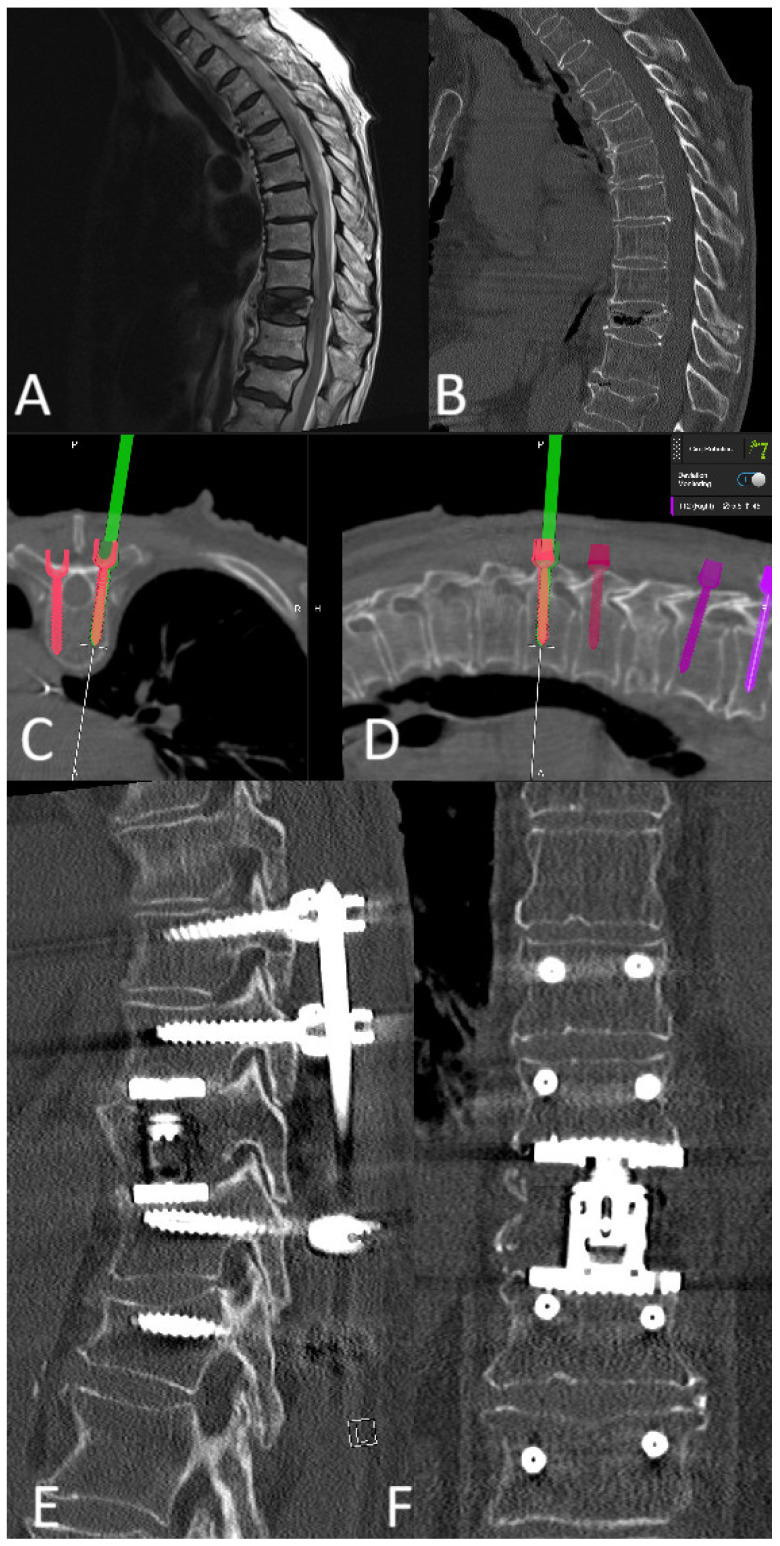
Preoperative (A) T2-weighted MRI and (B) CT of the thoracic spine, showing a pathological fracture of Th10 with instability. The patient underwent RG placement of K-wires followed by navigated placement of PS, shown here in intraoperative (C) axial and (D) sagittal view. In addition, an expandable vertebral body cage was implanted via a left transthoracic approach. Postoperative CT in (E) sagittal and (F) coronal view.
